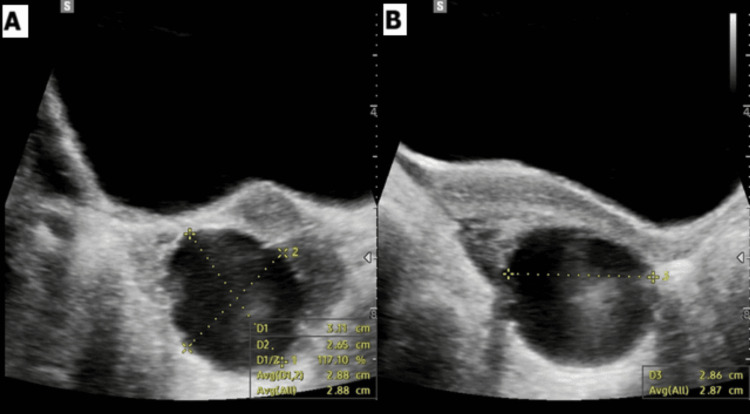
Ultrasound image demonstrating the left ovarian simple cyst in (A) transverse and (B) longitudinal views
